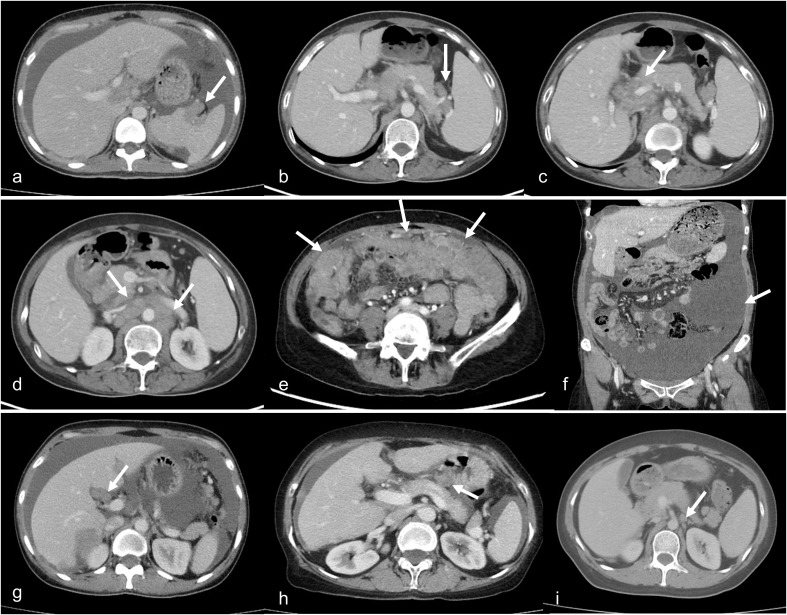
CT features assessed as part of the Suidan scoring system. Representative CT images demonstrating the radiologic parameters used in the Suidan score evaluation.(a) Lesion in splenic hilum/ligaments; (b) Lesion in splenic ligaments; (c) Porta hepatis lesion; (d) Retroperitoneal lymph nodes above the renal hilum; (e) Diffuse small bowel adhesions/thickening; (f) Abdominal ascites (moderate-severe); (g) Gallbladder fossa/Liver intersegmental fissure lesion; (h) Lesser sac lesion >1cm; (i) Root of the superior mesenteric artery lesion.
